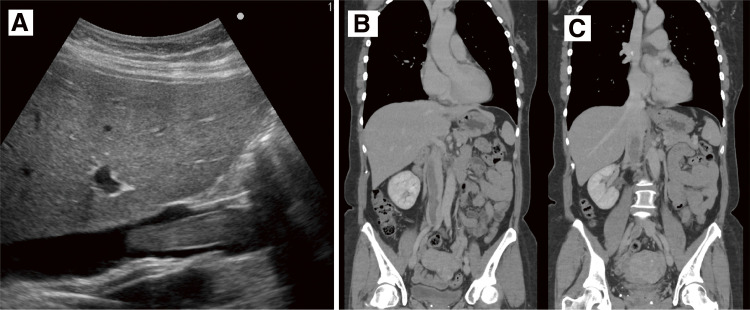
Fig. 3 (A) The abdominal ultrasound test revealed a suspicion of tumor growth in size. (B, C) At the 6-month follow-up CT, the cranial portion of the tumor had grown within the IVC, reaching below the right hepatic vein, raising suspicion of tumor enlargement. IVC: inferior vena cava
